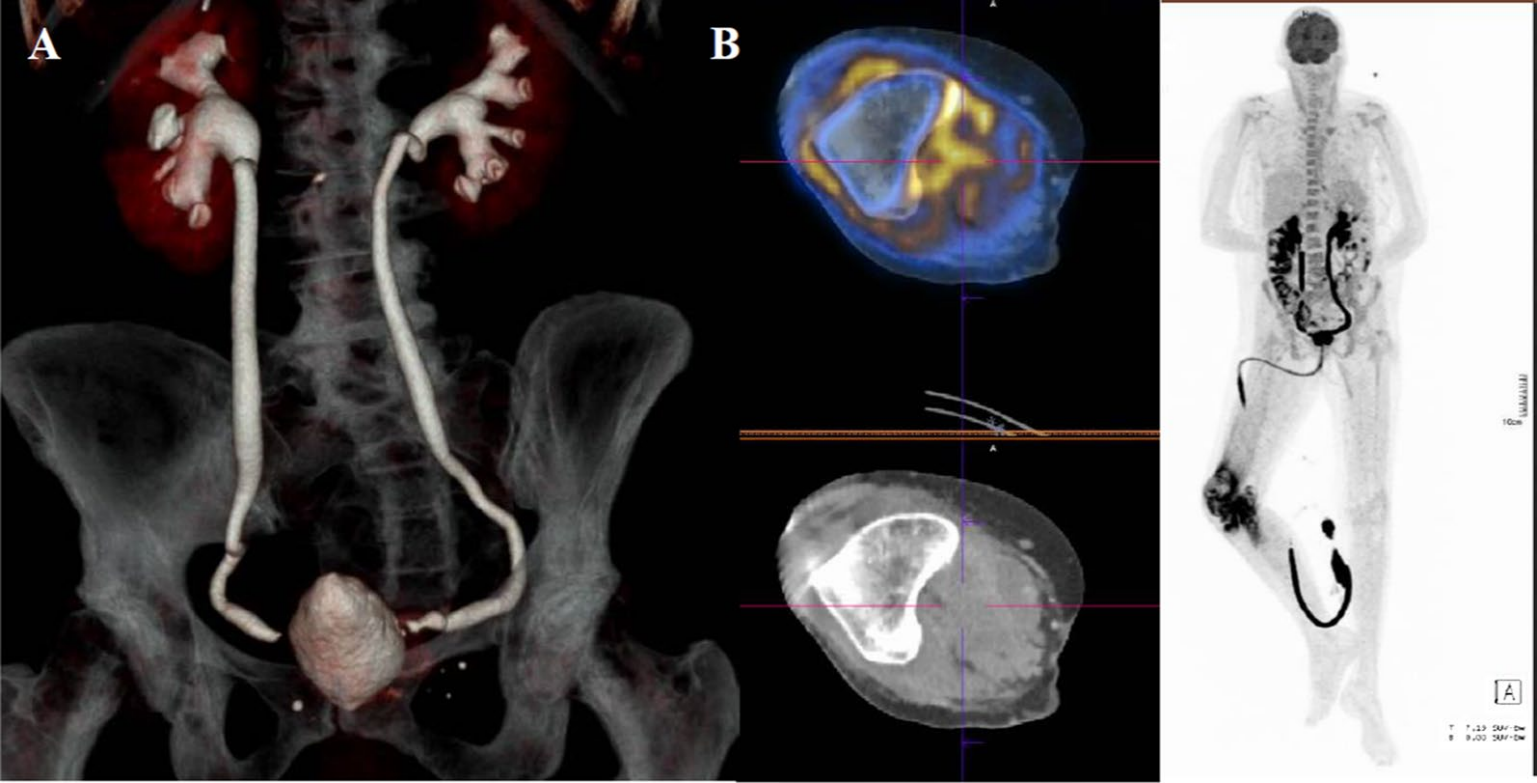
a Positron emission tomography tomodensitometry showing hypermetabolism of the right knee (maximal standardized uptake value [SUVmax]: 11.8) and right latero-urine hypertabolism (SUVmax: 7.5). b Computed tomography showing dilatation of urinary tract and bilateral ureteral stenosis
